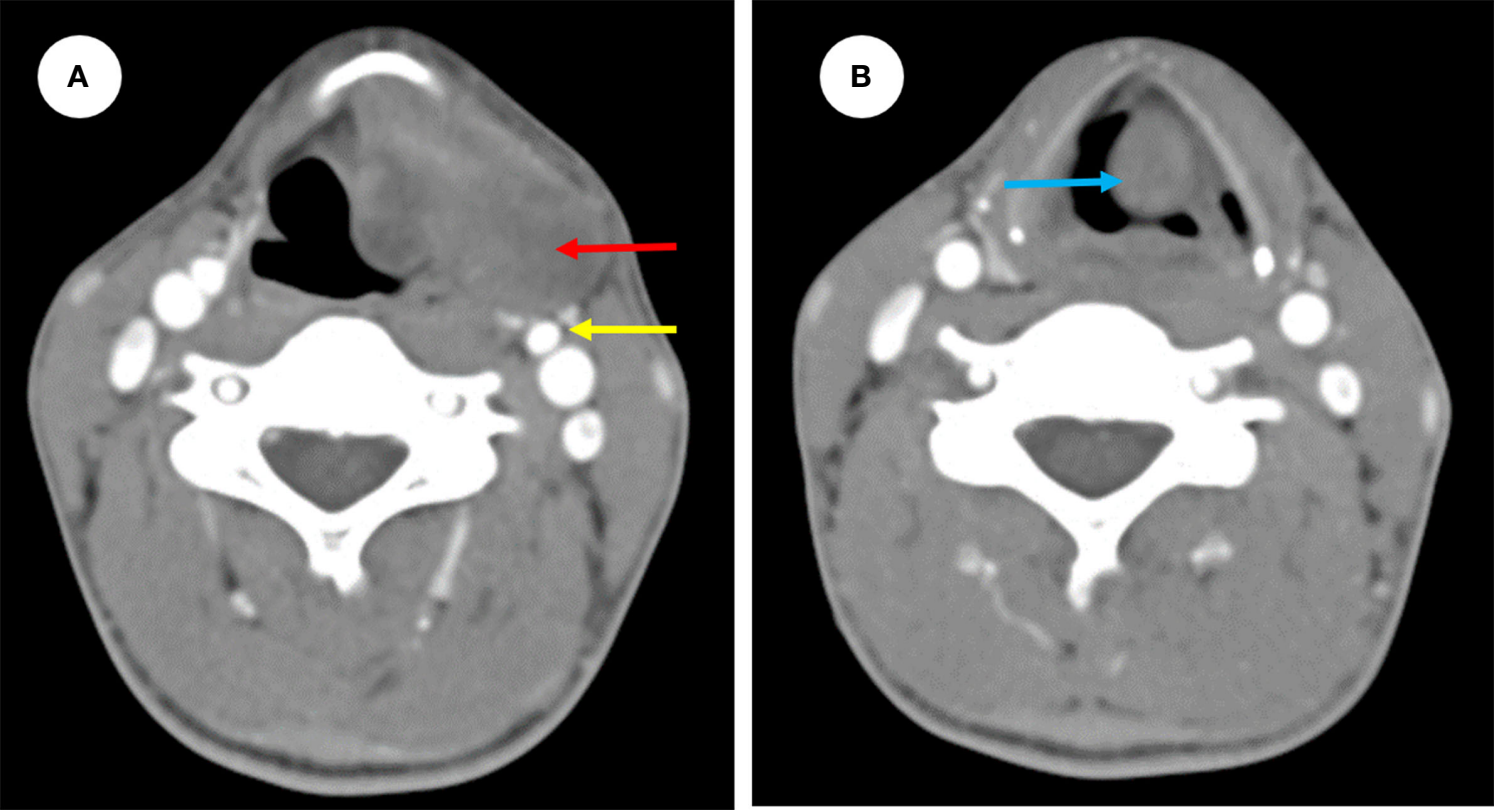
Preoperative enhanced CT images showing a mass of irregular density (red arrow) located anterior to the carotid (yellow arrow) extending into both the left side of preepiglottic space (red arrow) (A) and the laryngeal cavity (blue arrow) (B).
